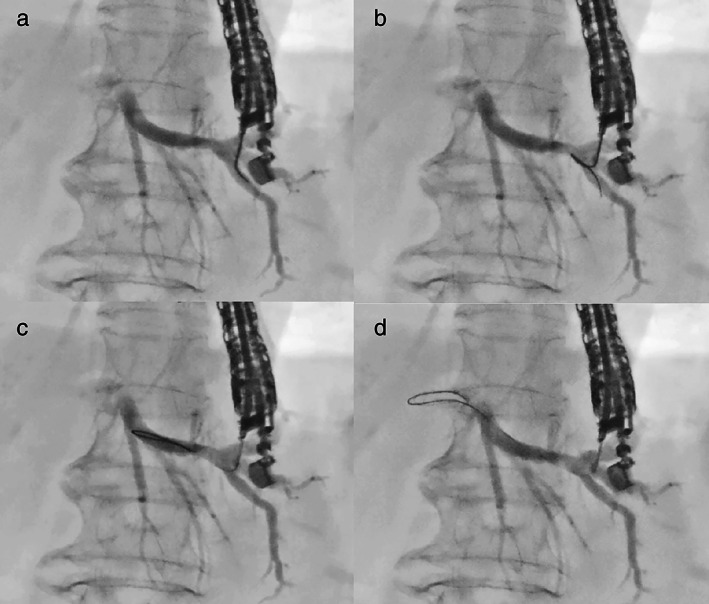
Endoscopic ultrasound‐guided hepaticogastrostomy with loop technique. (a) The guidewire is initially advanced toward the peripheral side. (b) The tip of guidewire is inverted. (c) Looped guidewire is advanced toward the hilar side. (d) The guidewire has successfully reached the left hepatic duct.
